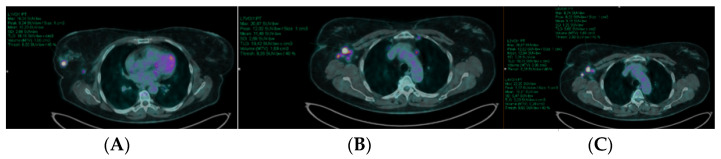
Image analysis is presented in the figure (transaxial fused images of a patient performing baseline [^18^F]FDG PET/CT). Breast primary tumor (A) and the most representative axillary lymph node metastasis (B) were contoured with a circular ROI. The system automatically adapted the ROI into a 3-d VOI and calculated semiquantitative parameters as shown in the figure and reported in the text. Moreover, all lesions showing increased [^18^F]FDG uptake were contoured (C) to obtain the whole body load of disease, in terms of MTV_WB and TLG_WB.
